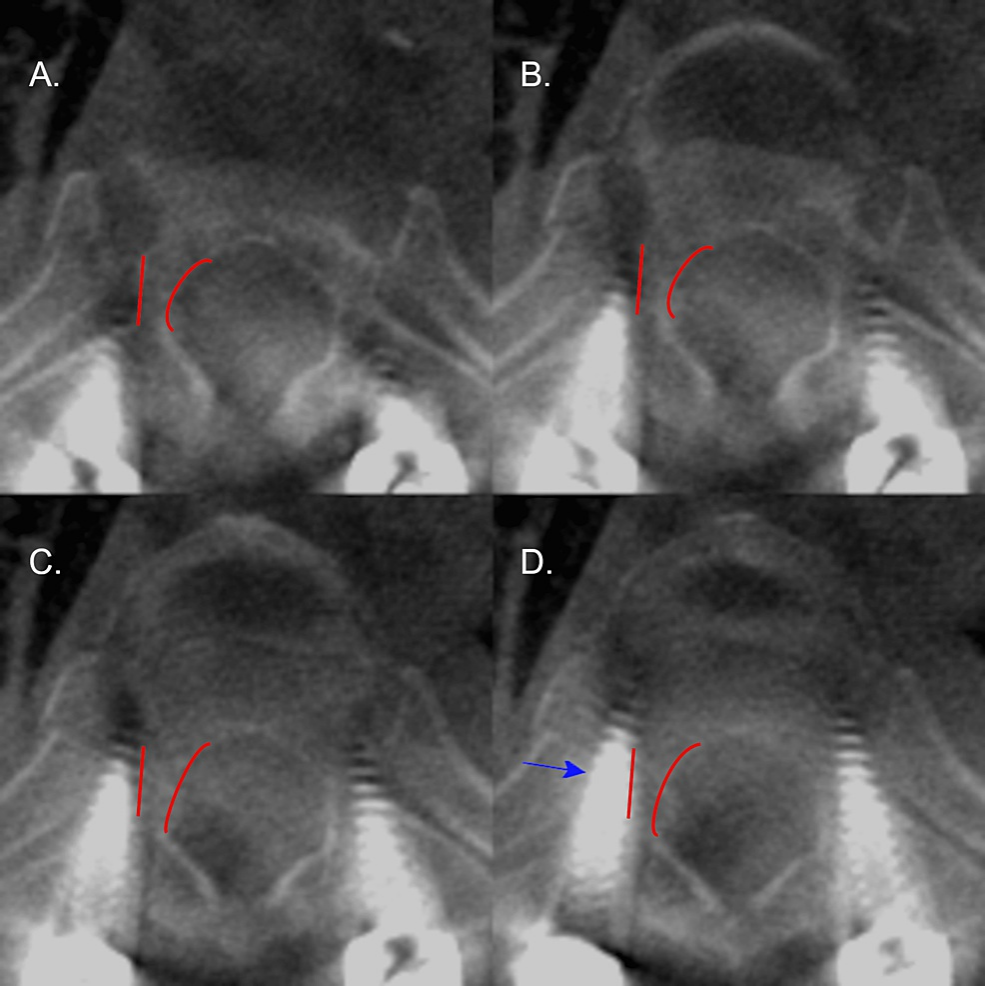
Axial CT radiographs of the right T6 pedicle screw on patient 13 Axial CT radiographs demonstrating the right T6 pedicle screw placement on patient 13 with an in-out-in (IOI) trajectory. Screw placement progresses from A to D. The pedicle of interest is outlined in red. The blue arrow denotes the largest deviation of the screw. Please note all CT images are in radiographic orientation.
